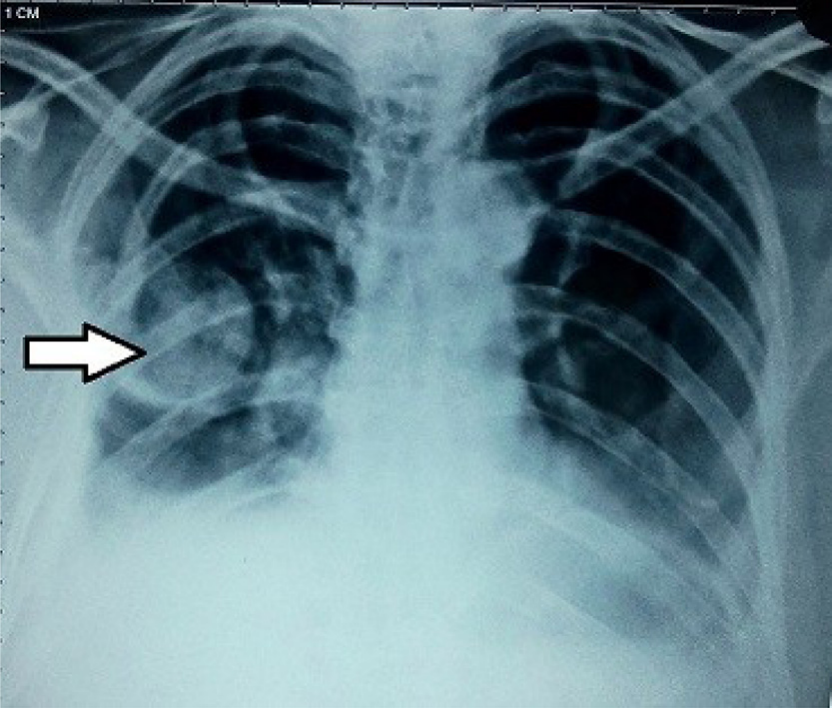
X-ray showing a well defined circular opacity in middle zone of right lung.
